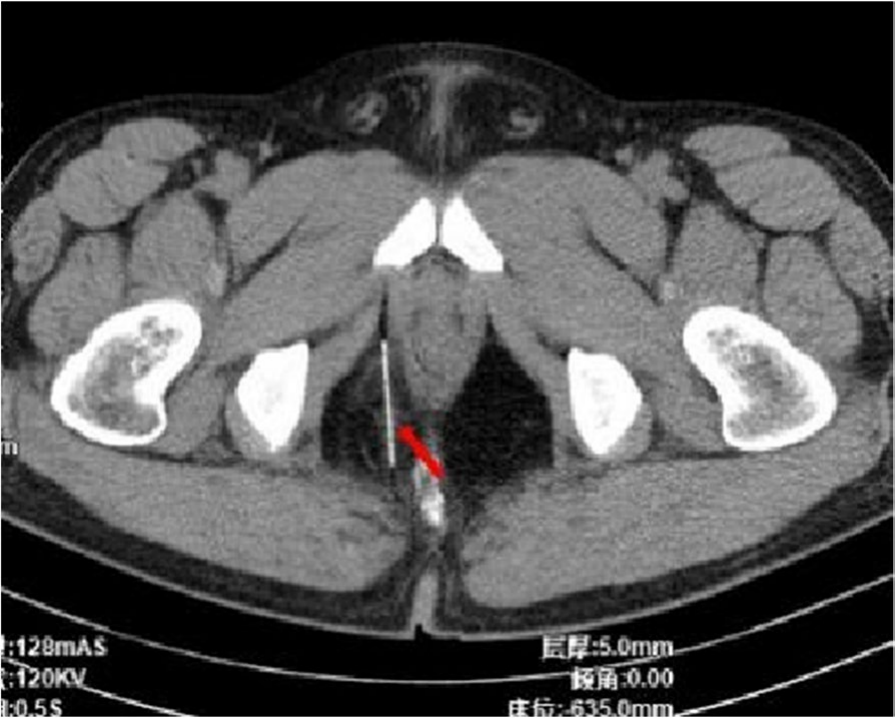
August 7, 2023, CT scan of the pelvis. The arrow points to the residual needle
